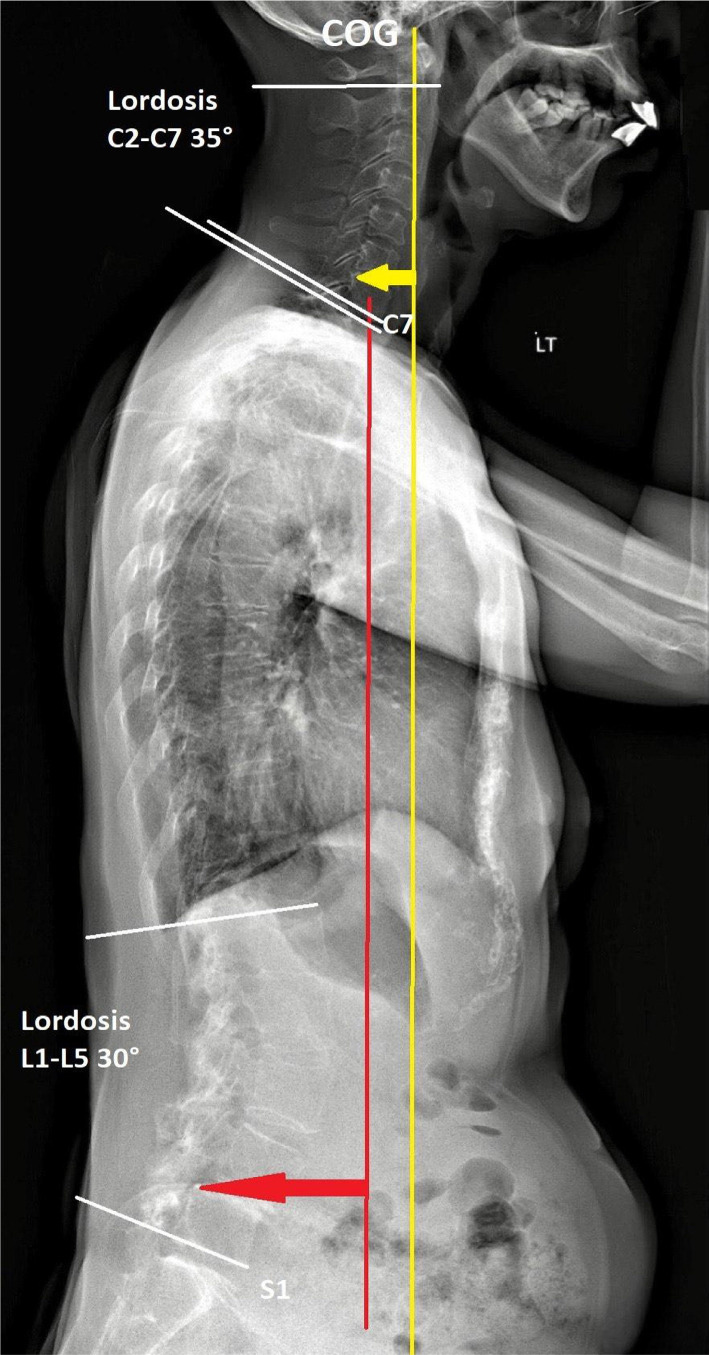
A repeat radiograph taken nine months later demonstrates significant improvement in the majority of the spinal deformity. The C7 plumb line (red line) is drawn caudally from the C7 vertebra's center. The line should be parallel to or within 5 mm of S1's superior-posterior endplate. In a well-aligned subject, the line of COG (yellow line) has improved in the sagittal plane.
